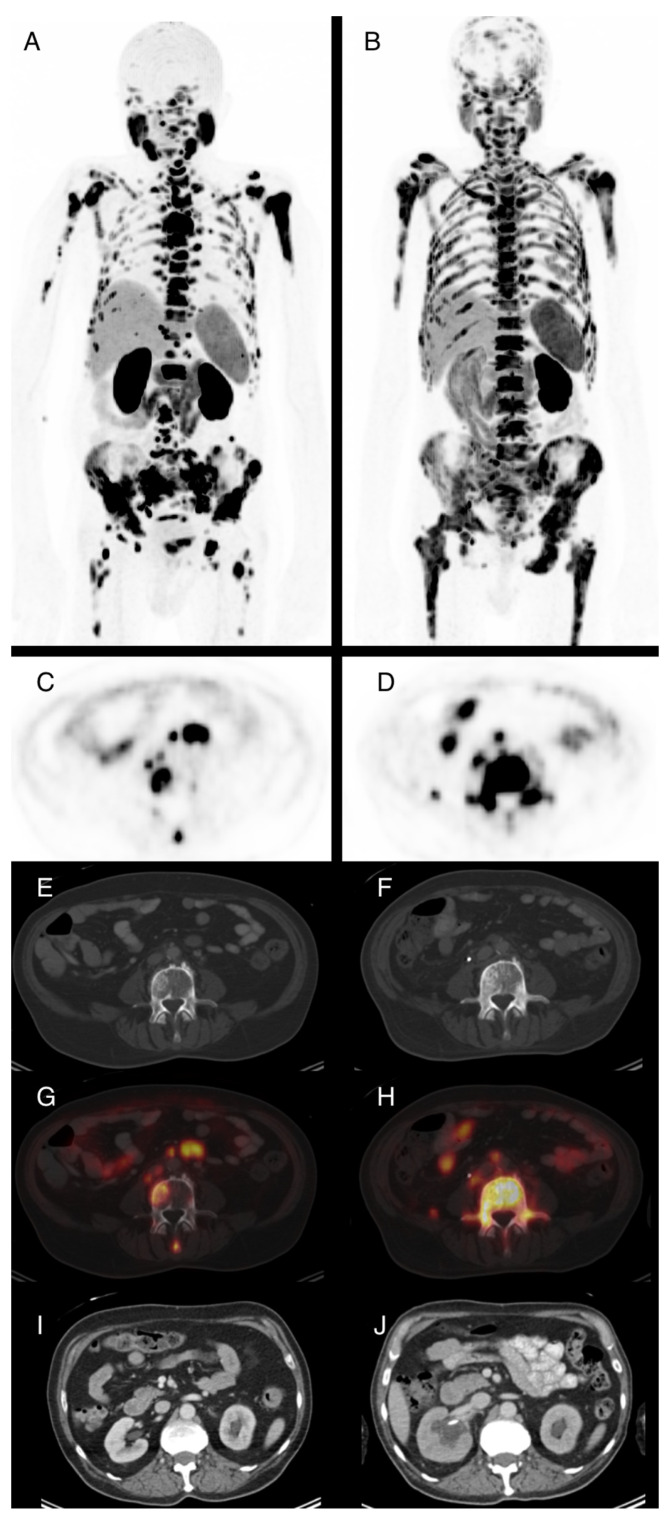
74-year-old patient with locally recurrent prostate cancer and metastases in lymph nodes and bone. Maximum intensity projections of F-18-PSMA-PET/CT before (A) and after ((B), SUVmax. 15) two cycles of Lu-177-PSMA radioligand therapy (RLT) with application of 10.7 GBq total demonstrate progressive disease. PSA levels were 169 ng/mL prior to RLT and 233 ng/mL after two cycles. Axial PET images show increasing PSMA-expression in the L4 vertebra (C,D) that is not totally reflected in corresponding CT images with sclerotic bone lesions (E,F). (G,H) show image fusion of PET and CT at the same position as (E,F). Due to progressive metastatic obstruction of the right ureter, postrenal kidney failure with hydronephroses occurred and a ureteral stent was placed (J, compared to I). This also explains the reduced tracer excretion via the right kidney observed in (B).
